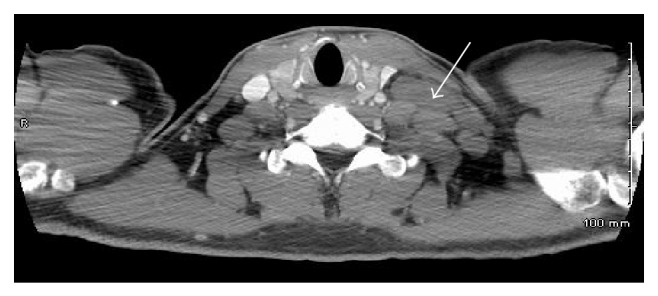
CT scan of the neck with left supraclavicular lymphadenopathy (arrow).
